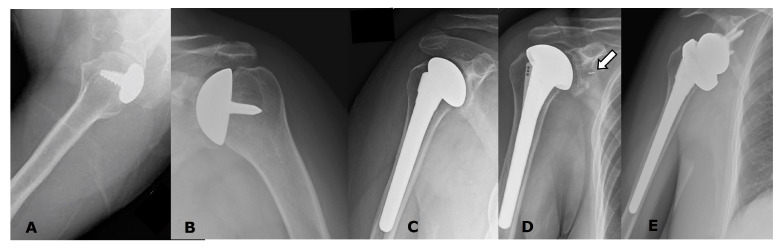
Radiographs of several different uncomplicated arthroplasty types. (A). Partial humeral head resurfacing arthroplasty (HHRA). (B). Humeral head resurfacing arthroplasty (HHRA). (C). Hemiarthroplasty (HA). (D). ATSA. Note small radiopaque marker identifying the glenoid component (arrow). (E). RTSA.
